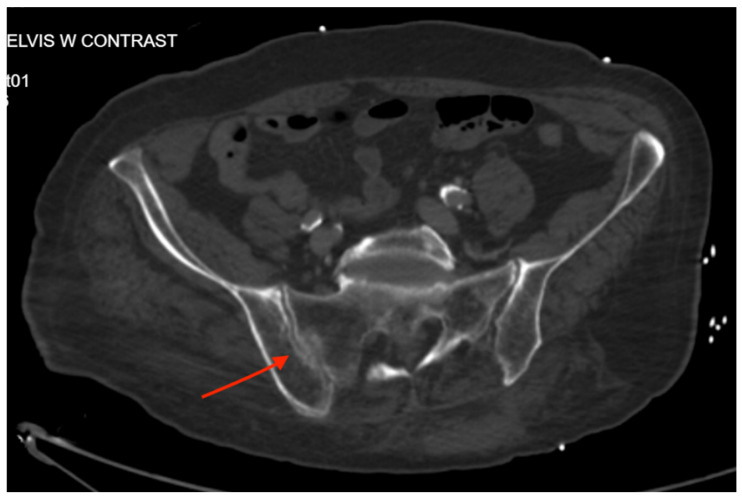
CT abdomen/pelvis images of the described fracture in case 6. Red arrows are used to indicate the location of pelvic fractures for easier identification.
